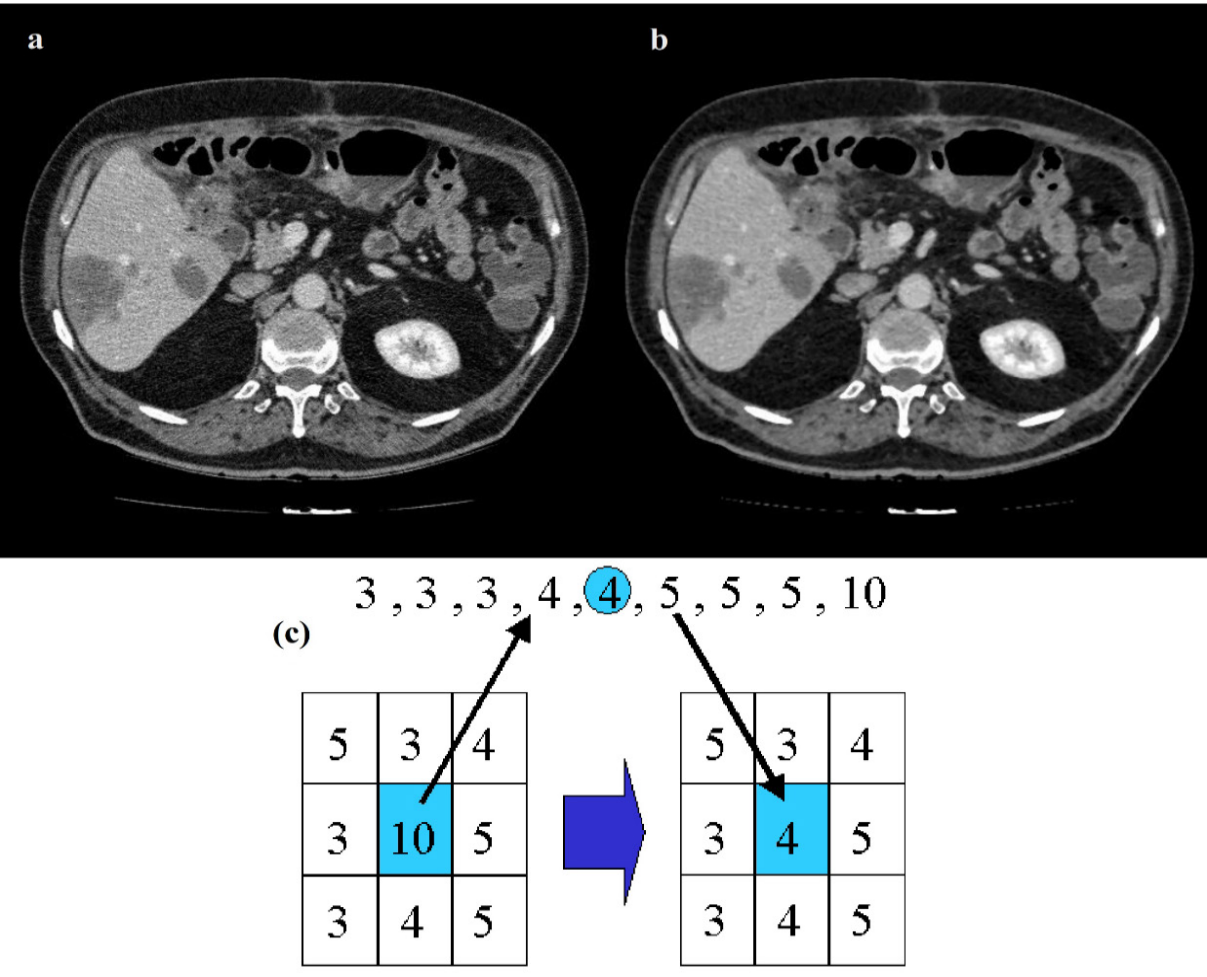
Results of median filtering, original image (a) applied to a CT image (b), median filter (c)
